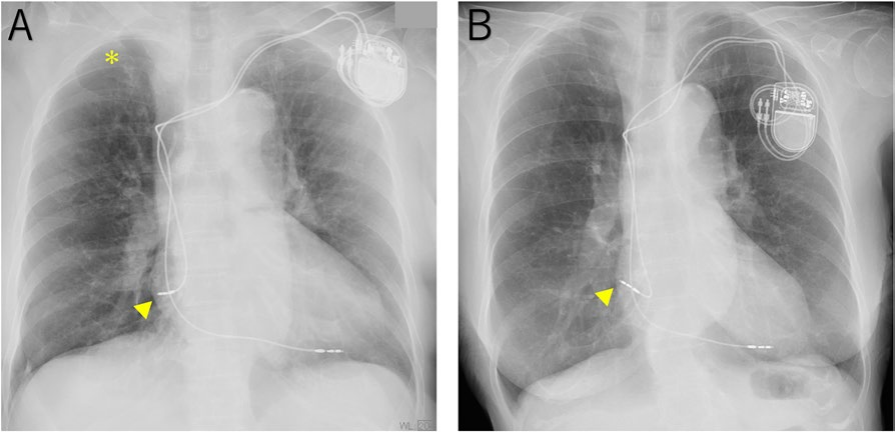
Chest X-ray images. A X-ray images taken in the sitting position on admission. The asterisk (✻) indicates the area of the collapsed lungs. The yellow arrowhead indicates the tip of the lead. B X-ray images were taken immediately after pacemaker implantation. The yellow arrowhead indicates the tip of the lead. The position of the tip has migrated
